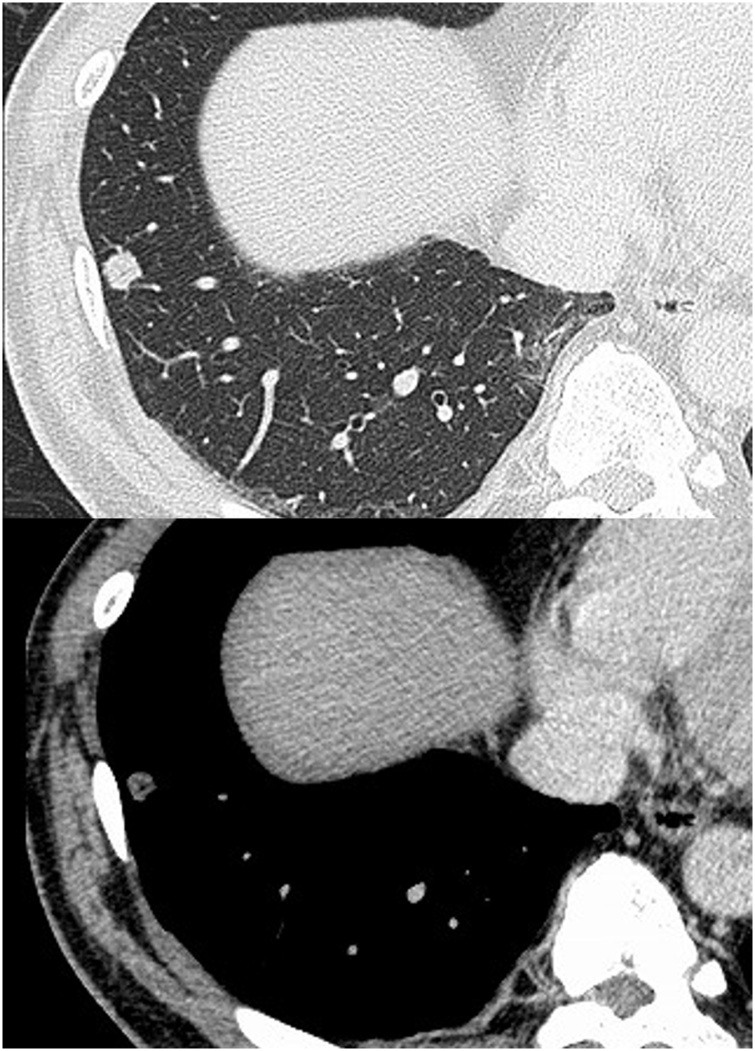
Radiological findings. Computed tomography showed a well-circumscribed, 9-mm mass in the S8 segment of the right lower lung lobe.
